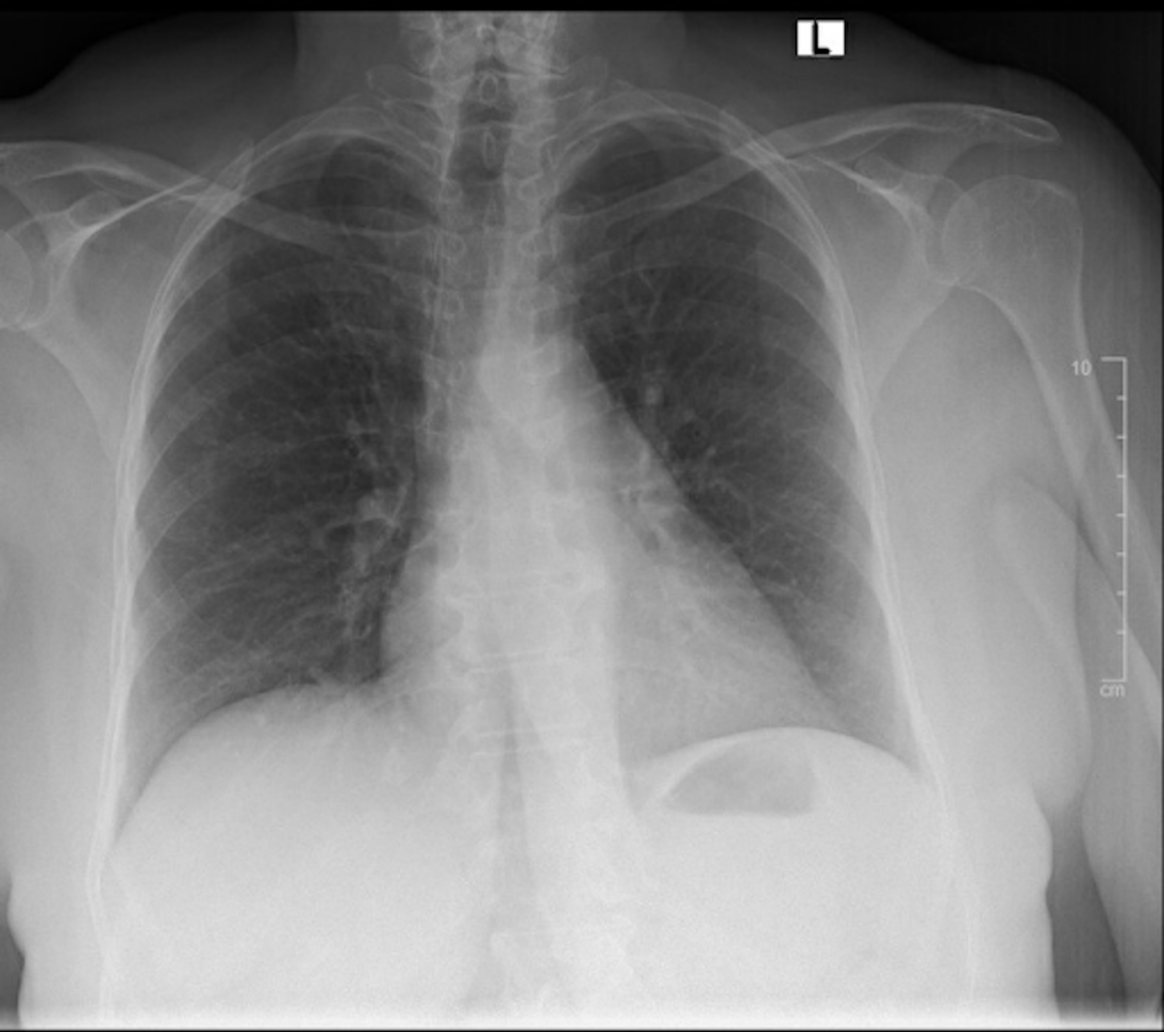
Previous chest X-ray from 2018 showing no acute pulmonary disease
